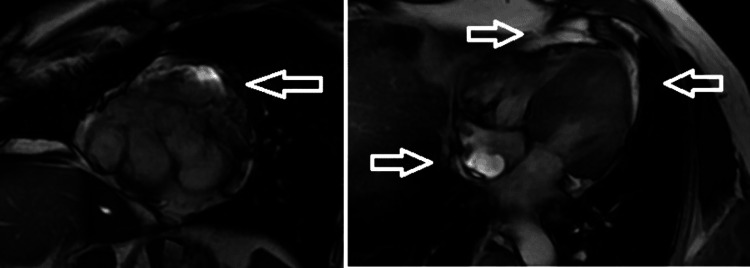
Cardiac MRI showing a delayed gadolinium enhancement suggestive of infiltrative disease MRI: magnetic resonance imaging
